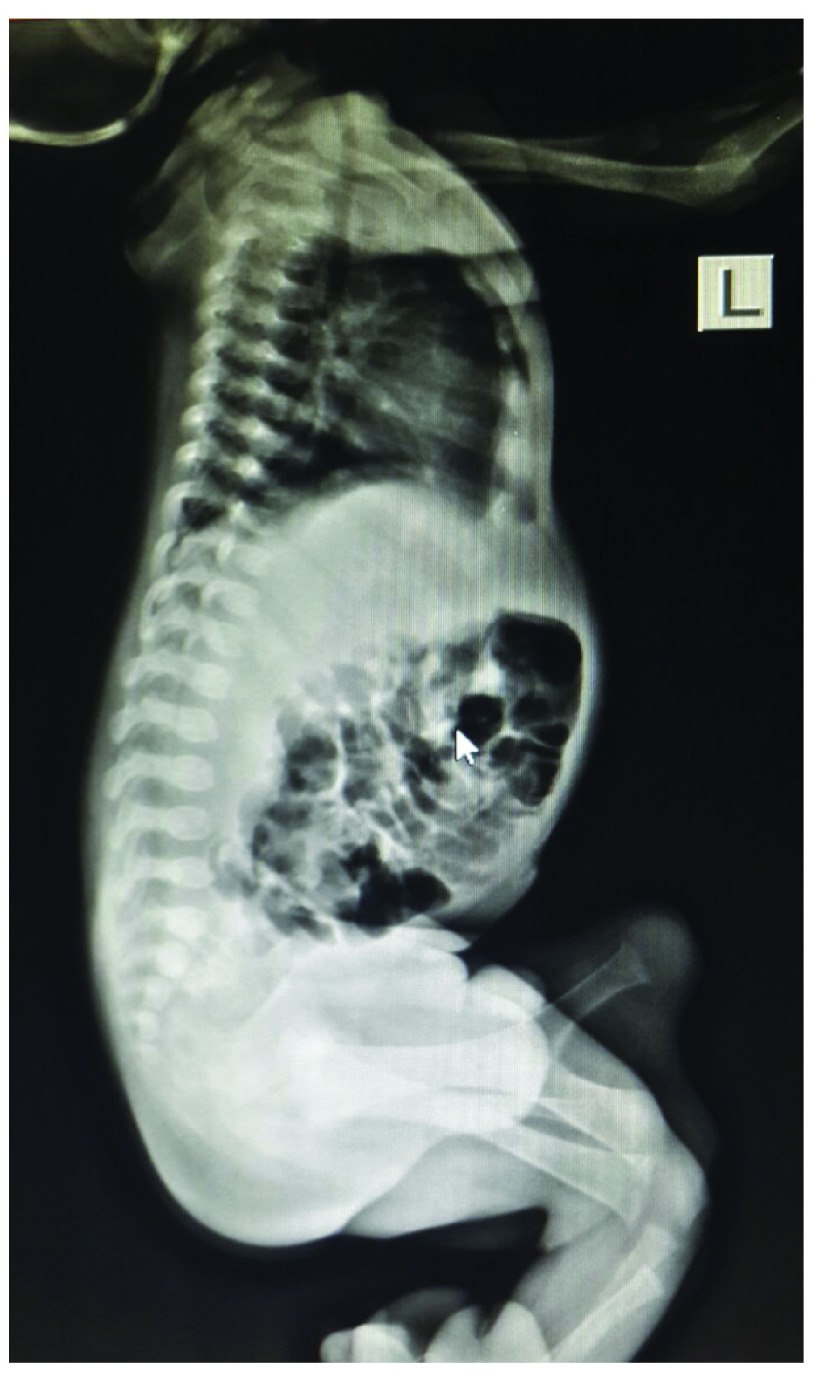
X-ray showing caudal (sacrococcygeal) agenesis.
